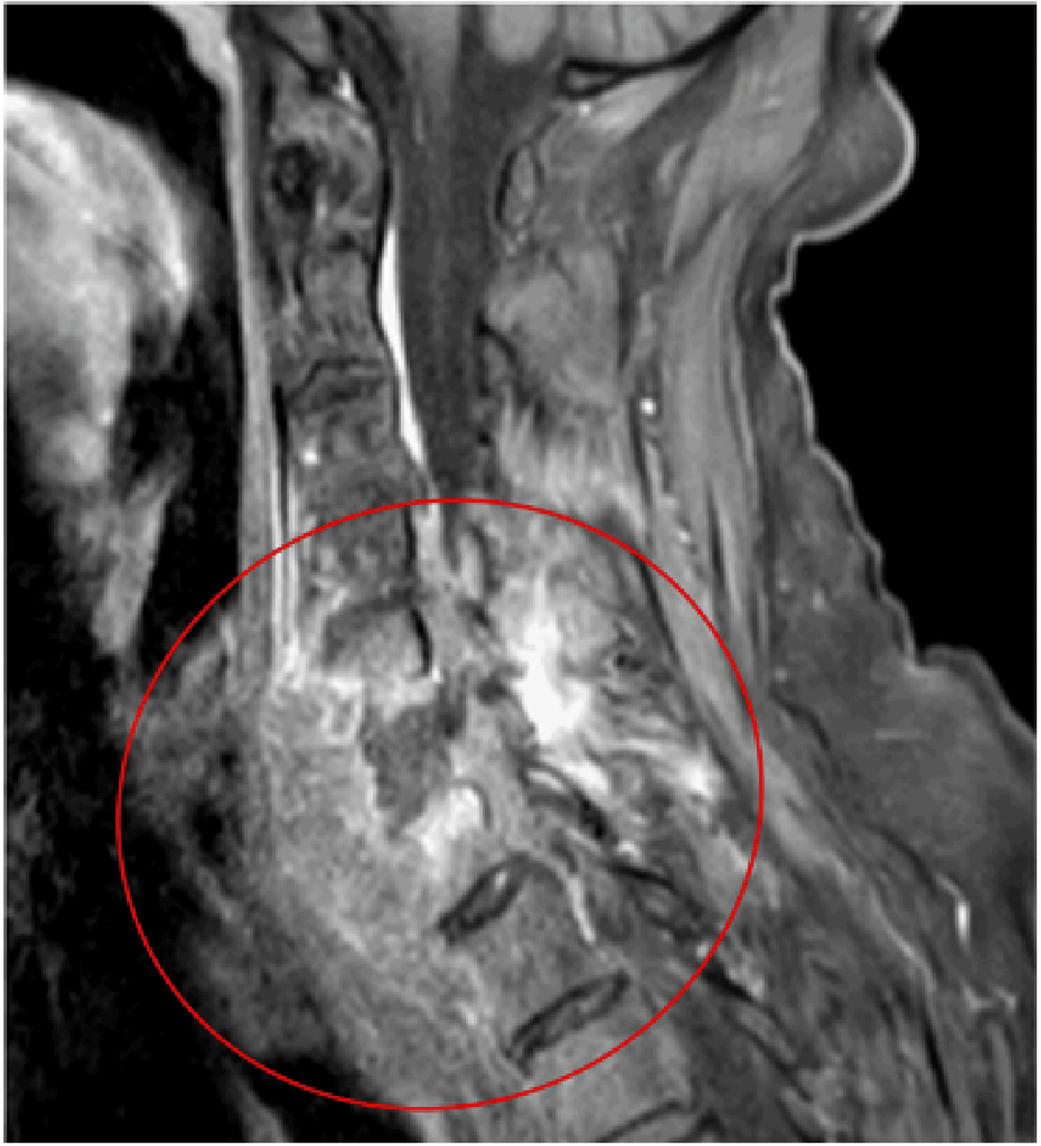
MRI of the cervical spine confirming severe spinal cord compression due to the lesion presente in the C6 vertebra measuring approximately 6.6x2.6 cm and extending from C5 to T1, causing compressive myelopathy.
